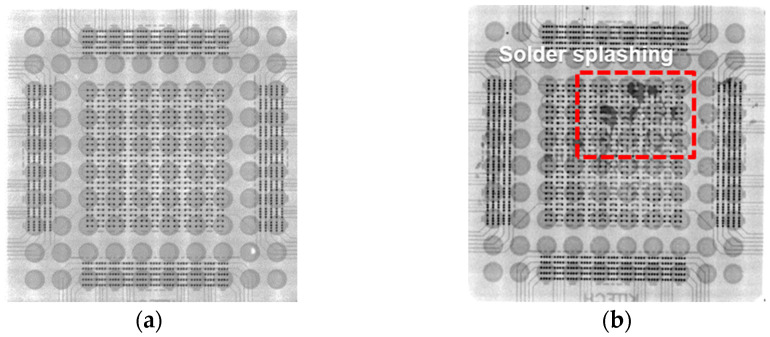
X-ray image of the SnAg/Cu pillar bump joints with a laser power density of 2.8 W/mm^2^. The laser irradiation time conditions were (a) 1.0 s and (b) 1.9 s.
